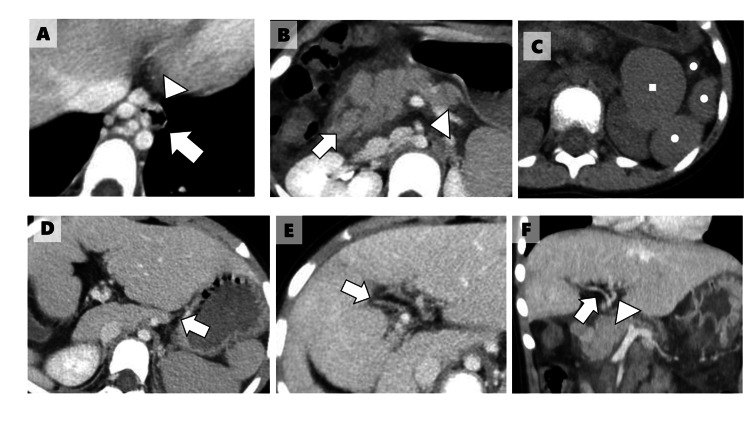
Dynamic contrast-enhanced CT A. Esophageal varices extend from the thoracic to the abdominal esophagus. No extravasation of contrast agent is observed (arrow: esophagus; arrowhead: esophageal varix). B. The horizontal part of the duodenum is located far from the superior mesenteric artery, indicating intestinal malrotation (arrow: horizontal part of the duodenum; arrowhead: superior mesenteric artery). C. Multiple spleens are observed (circle: spleen; square: stomach). D. Agenesis of the pancreatic tail is observed (arrow: pancreatic tail). E and F. The portal vein is occluded just proximal to its entry into the liver (arrow: portal vein; arrowhead: hepatic artery). The intrahepatic portal vein is poorly enhanced. CT: computed tomography
